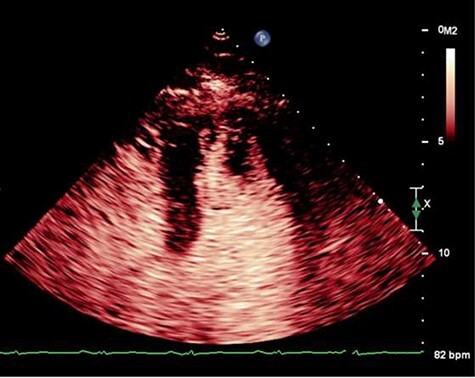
Pre-operative echocardiogram showing LV thrombus.
